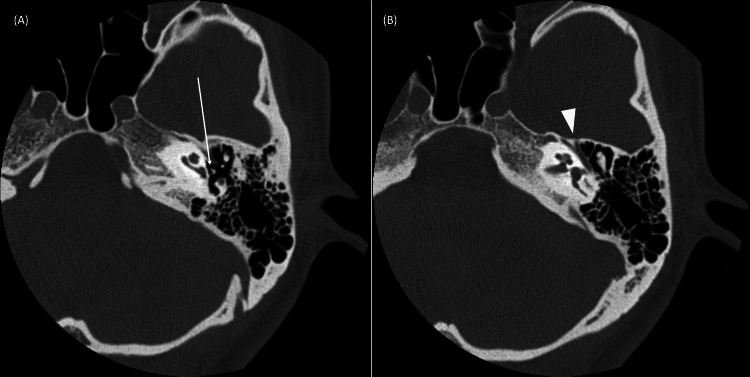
Temporal horizontal bone CT scan of the left temporal bone. (A) The white arrow indicates a thicker anterior limb of the stapes. (B) The arrowhead indicates an abnormal facial nerve pathway.
